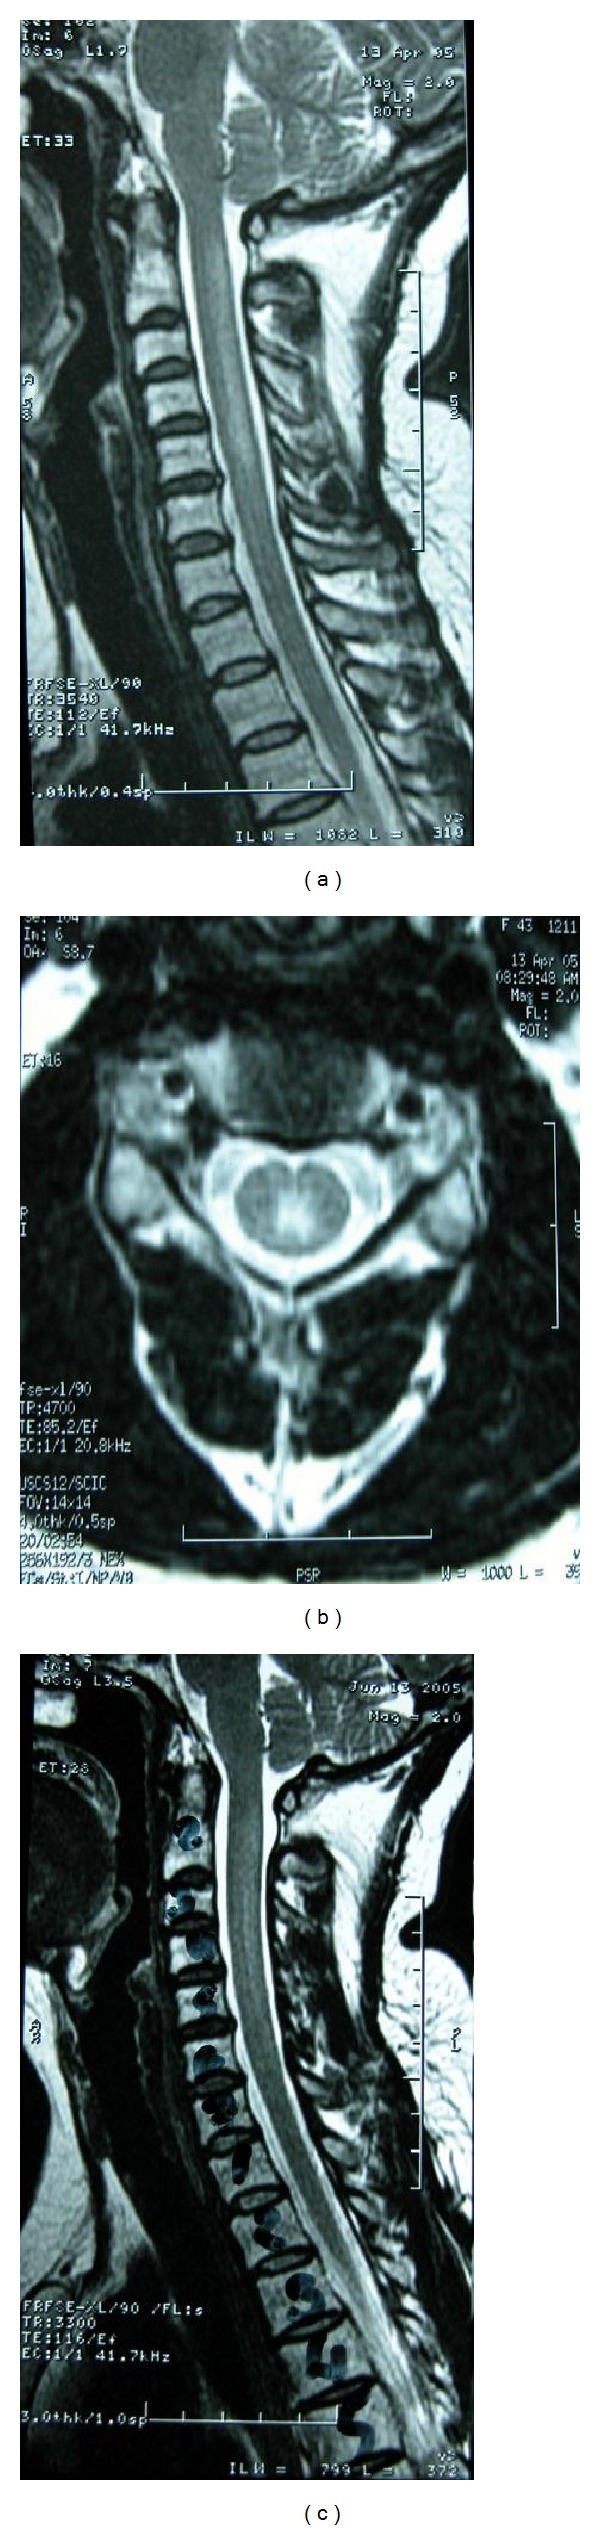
((a), (b)) Sagittal and axial T2 weighted images of the cervical cord before treatment demonstrate high signal bilaterally in the posterior columns of the cord. (c) The posttreatment images 2 months later show a striking reduction of the MRI abnormalities.
